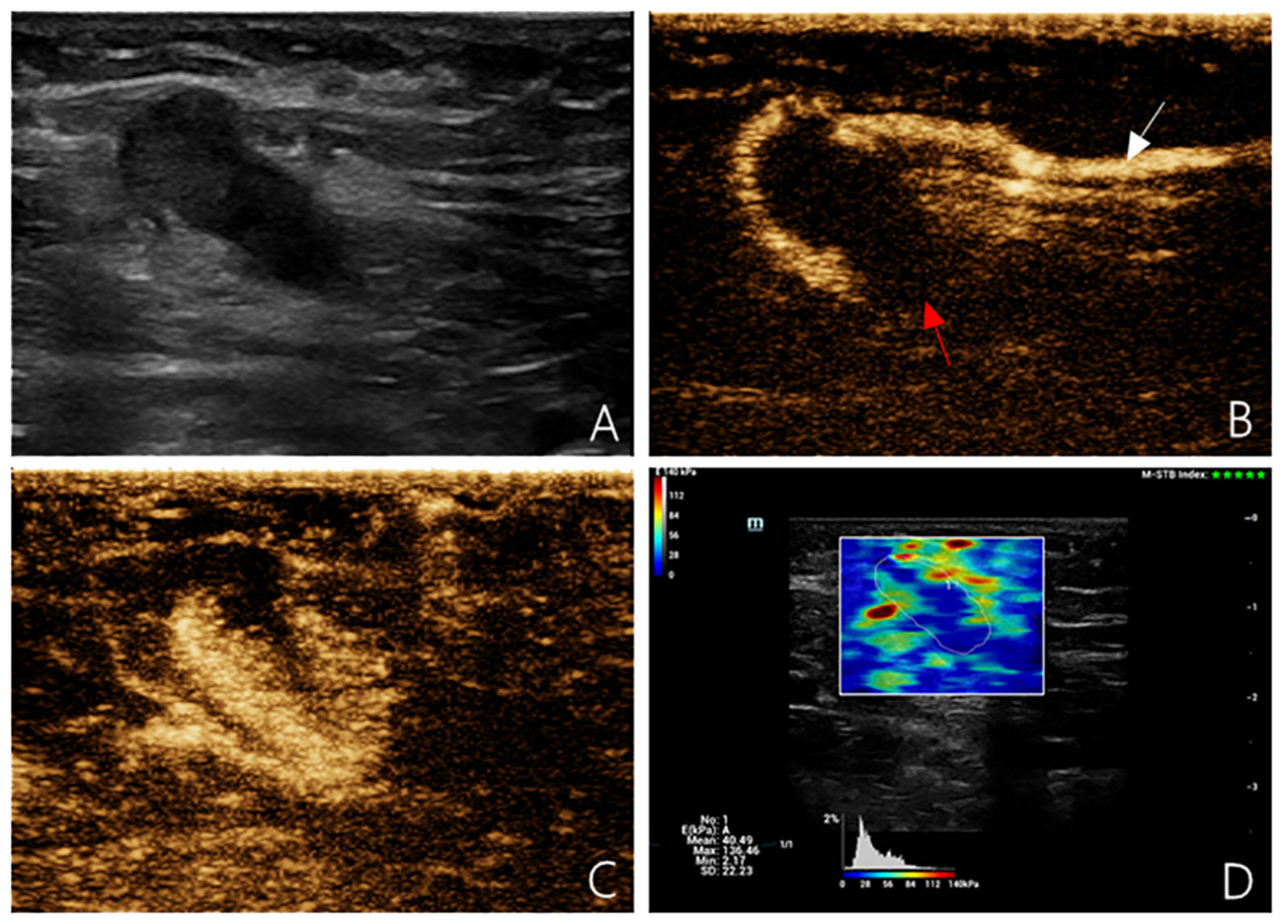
Multimodal ultrasound imaging of pathologically confirmed metastatic sentinel lymph node. (A) 2D US image; (B) Intradermal CEUS image (the enhancement pattern is type V, the white arrow indicates enhanced lymphatic duct, the red arrow indicates non-enhanced SLN); (C) Intravenous CEUS image (the enhancement pattern is centripetal); (D) SWE image (Emean=40.49 kPa).
